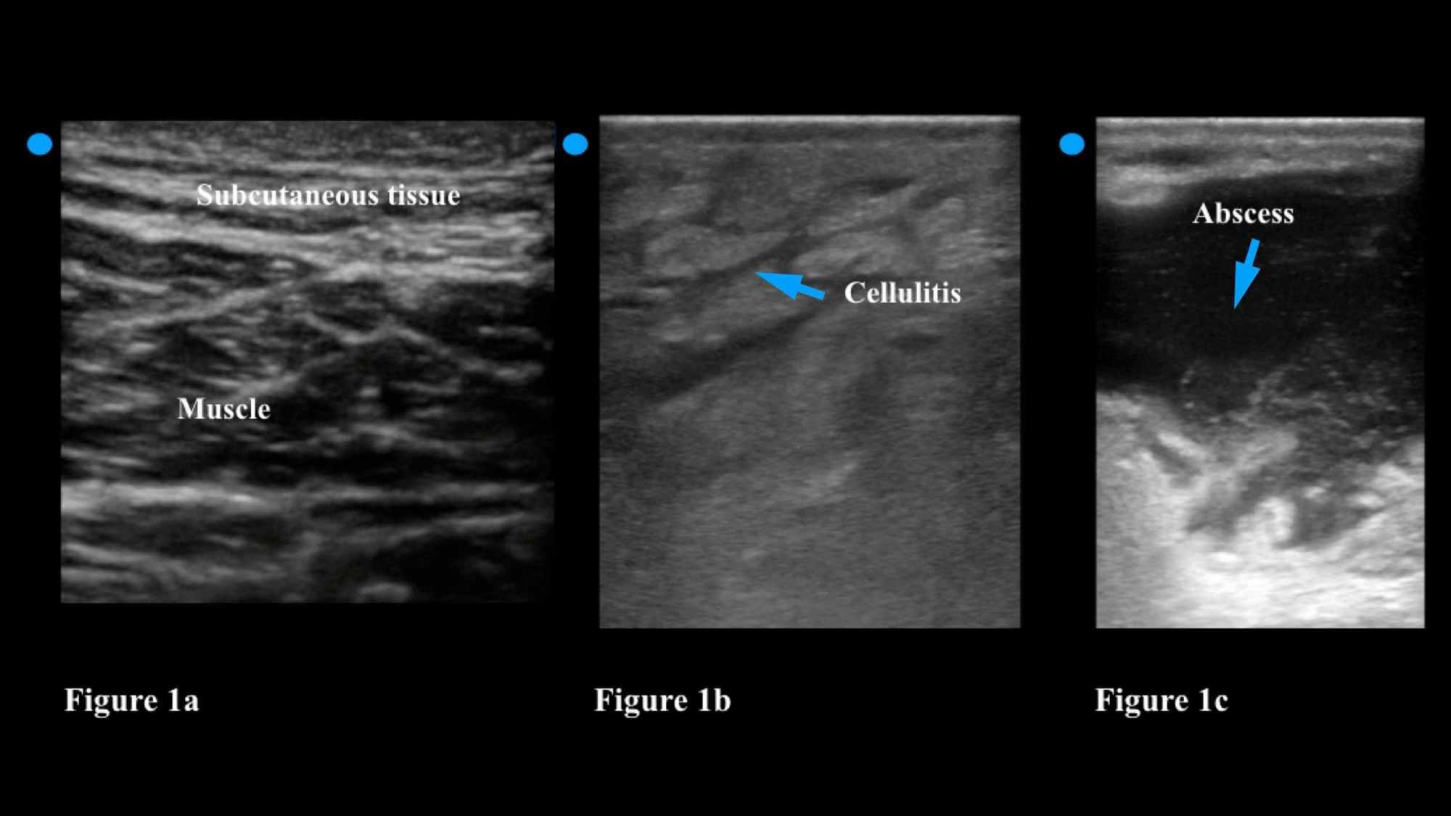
Figure 1a: Normal soft tissue ultrasound demonstrates thin layers of subcutaneous tissue overlying the muscle tissue. Figure 1b: Cellulitis is noted as thickening of the epidermis and subcutaneous layers; it is often described as ‘cobblestoning.’ Figure 1c: An abscess has a circumferential and usually irregularly shaped wall; it is filled with hypoechoic fluid and will often have echogenic particles floating within it.
